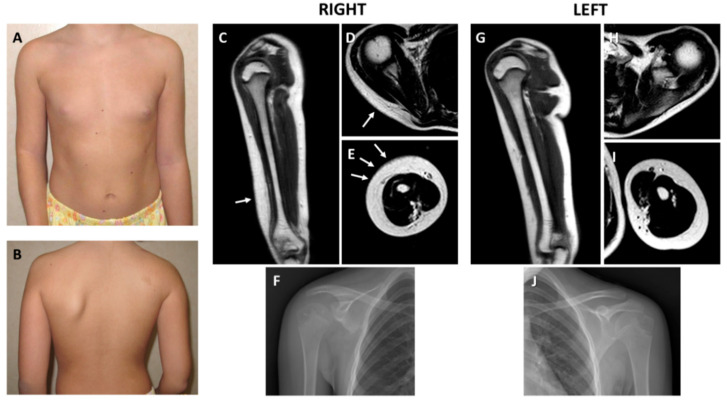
Clinical and radiologic features. (A) Front view showing limitation of the extension of the right elbow with accentuation of the cutaneous articular fold. (B) Back view demonstrating hypoplasia of the right scapula; note a small café-au-lait spot in the upper scapular region. Radiological findings of the right upper limb. (C) Thickening of the dermis of the lateral side of the arm at Magnetic Resonance Imaging (MRI) coronal view (arrow). (D) Thickened dermis at the scapular level (arrow). (E) Thickened dermis surrounding the arm muscles at axial view (arrows). (F) Plain radiograph documenting hypoplasia of the inferior part of the right scapula. Radiological findings of the left upper limb. Comparison with similar MRI sections of the affected (right) side demonstrated normal representation of the dermis at coronal (G), and upper (H) and lower (I) axial views. Muscles are underdeveloped in the right arm. (J) Normal left scapula at standard X-rays.
